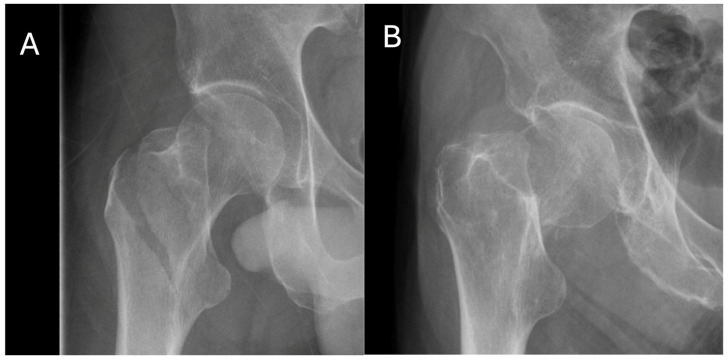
Radiographic images of the most common fracture types in the study population, according to the AO/OTA classification of femoral fractures. (A) 31A1.2, simple pertrochanteric two-part fracture, and (B) 31B1.3, femoral neck subcapital displaced fracture.
